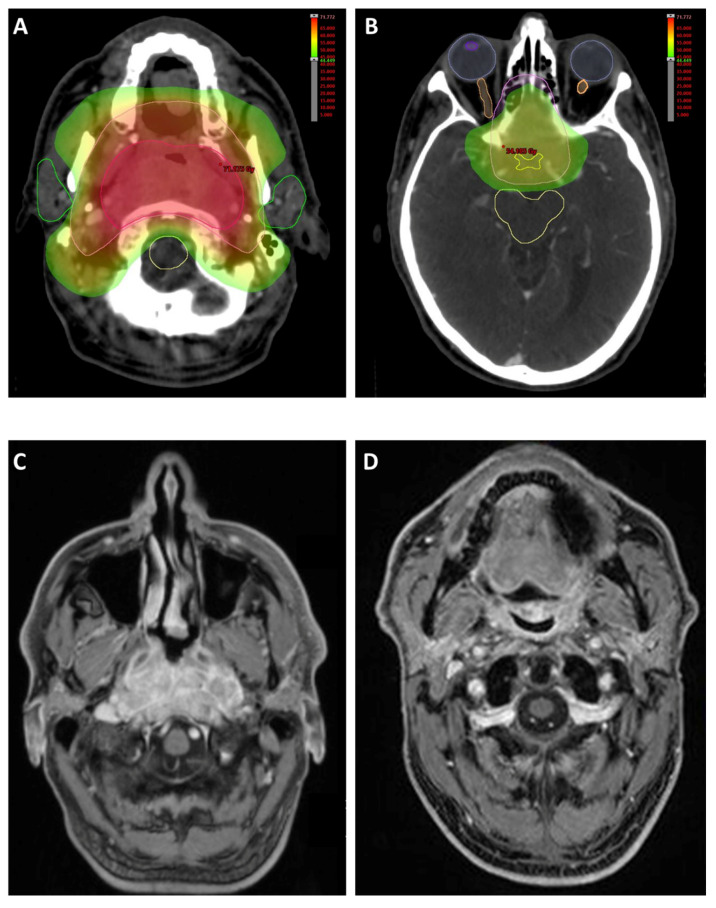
67-year-old male patient with an EBV-positive nasopharyngeal squamous cell carcinoma, infiltrating the sella turcica, clivus and right-sided internal carotid artery and affecting the chiasma, cT4 cN0 cM0, G3. Definitive cisplatin-based chemoradiotherapy was applied between May and July 2018. The primary tumor received 69.4 Gy in 37 fractions (14 fractions with 2 Gy and 23 fractions with 1.8 Gy). (A,B) Axial images showing bilateral parotid gland and spinal cord protection. (C) Contrast-enhanced MRI showing the nasopharyngeal carcinoma prior to radiotherapy. (D) Follow-up image at 2.5 years later (September 2020) showing no signs of recurrence.
